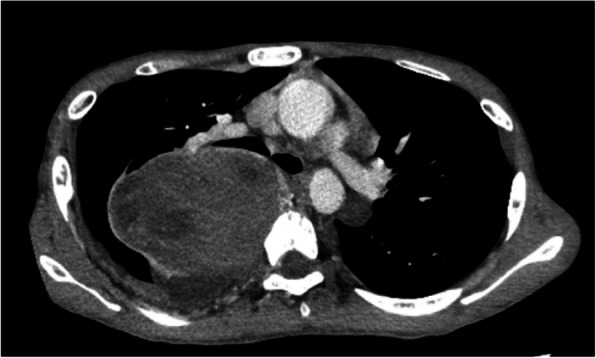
Preoperative computed tomography image at the level of the 5th thoracic vertebrae. Neurofibromas were not observed near the paravertebral space in this image
